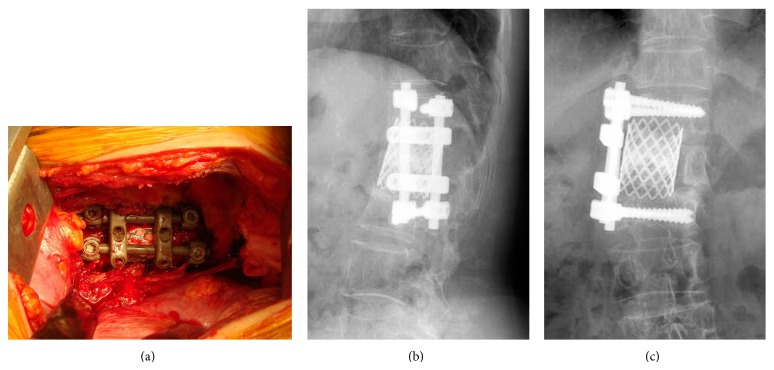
Anterior reconstruction from T12 to L2 using iodine-supported instrumentation. (a) Operative photograph. (Right side is directed cranially, and upper side is directed dorsally.) Postoperative radiographs of the lumbar spine. (b) Lateral view. (c) Anteroposterior view.
